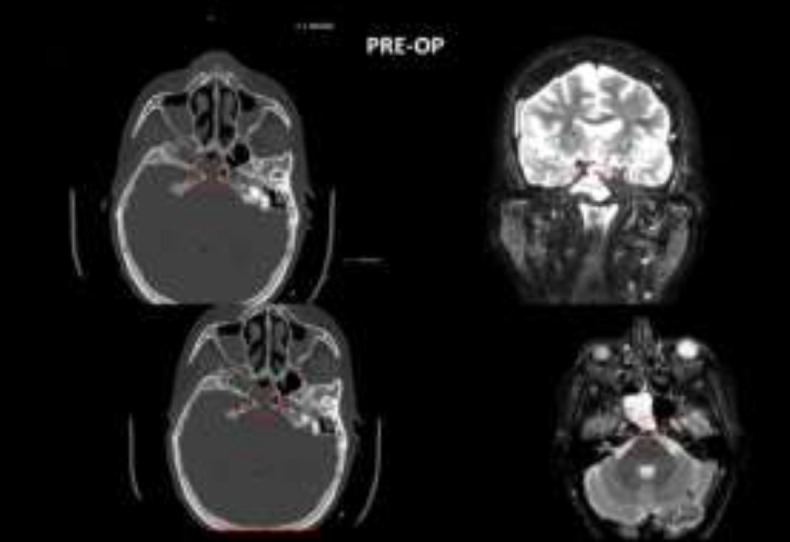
Clivus Fistula pre-op CT
